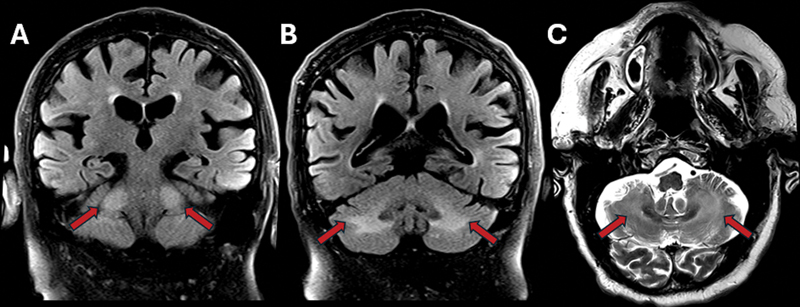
Brain magnetic resonance imaging (MRI) scan, coronal fluid-attenuated inversion recovery (FLAIR) (
